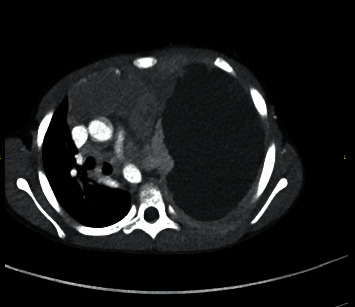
Chest computed tomography showing large right pleural effusion and anterior mediastinal mass with the mass effect.
